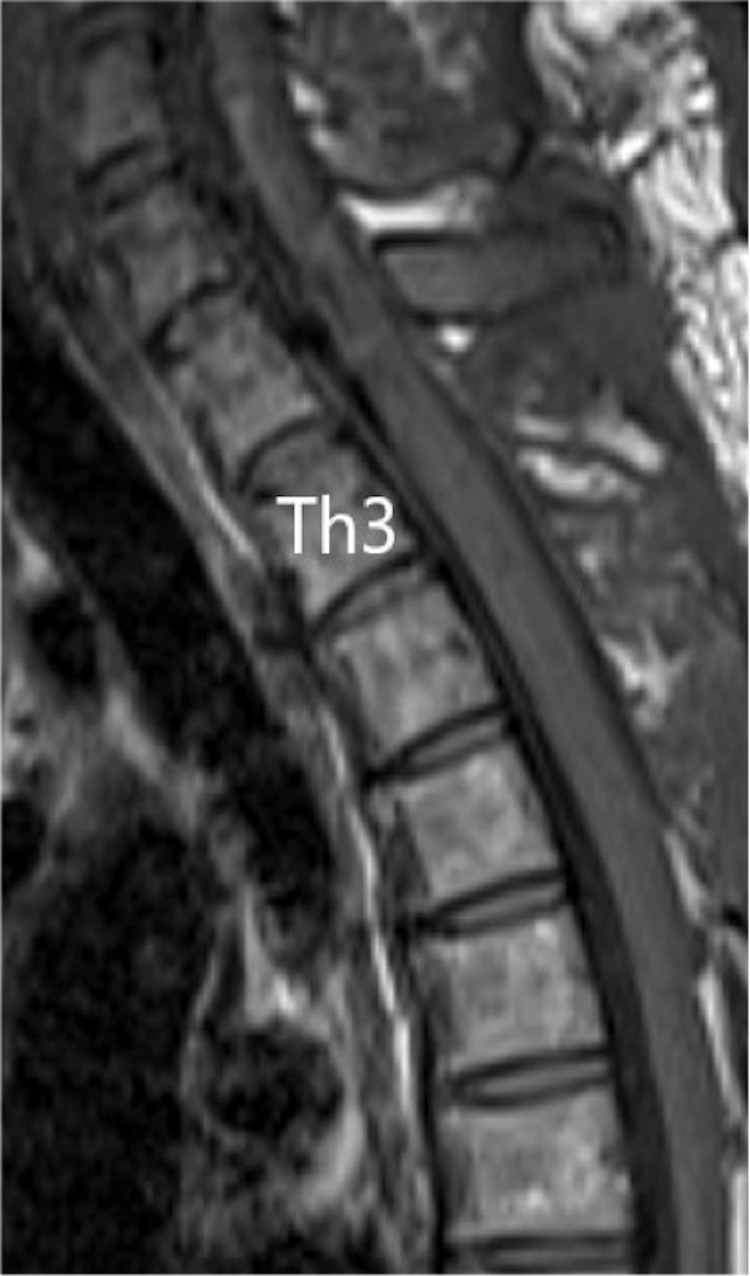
Sagittal T1-weighted MR images 12 weeks after the operation
